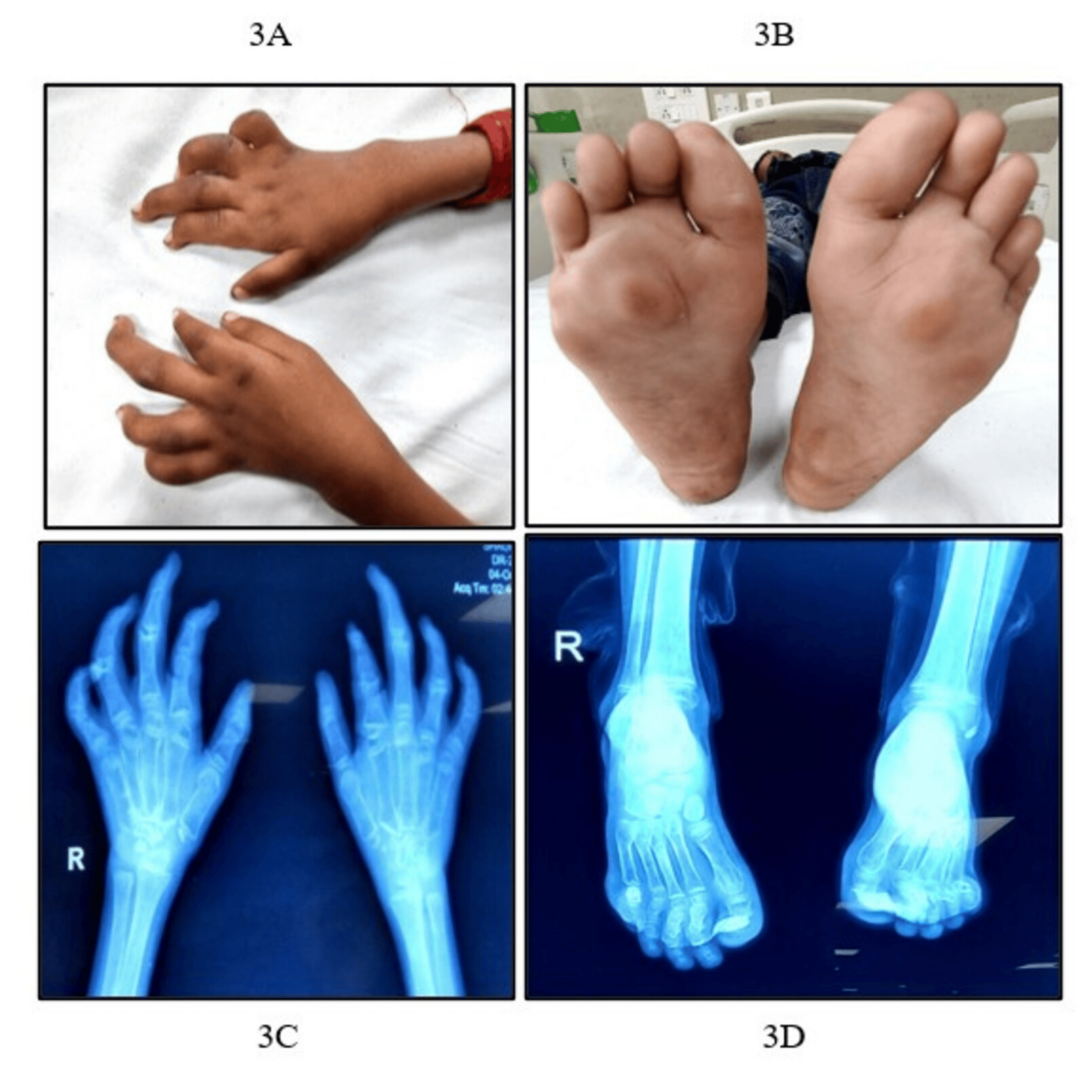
(A) Nodular swelling of MCP, PIP joints with contracture of PIP, DIP joints with wasting of thenar and hypothenar muscles. (B) Nodular swellings over bilateral plantar surface, hallux valgus, and contracture of the interphalangeal joints of the toes. (C) X-ray showing osteolysis of multiple carpal bones with flexion deformity of MCP, PIP, and DIP joints. (D) X-ray of the feet showing soft tissue swelling, diffuse osteopenia, and osteolysis of multiple tarsal bones with flexion deformity of the tarsometatarsal joints and IP joints. DIP, distal interphalangeal; IP, interphalangeal; MCP, metacarpophalangeal; PIP, proximal interphalangeal
